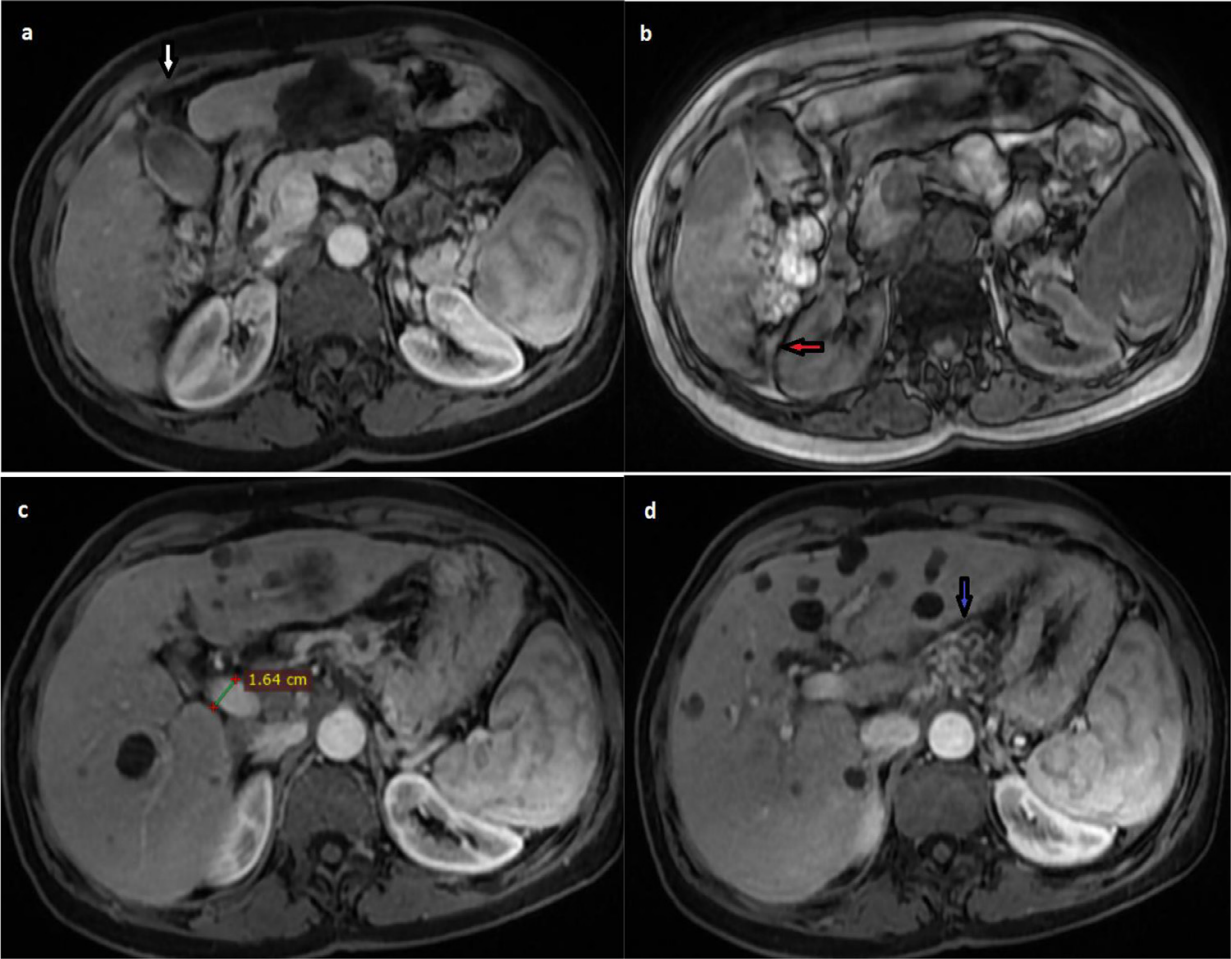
hepatic MRI on T1 post contrast WS (A, C, D) and OP WS (B) showing a cirrhotic liver with hypertrophy of the caudal lobe (note the right liver notch sign [red arrow), and atrophy of segment IV (white arrow), with portal hypertension (dilatation of the portal trunk and portosystemic collaterals [blue arrow]). (Color version of figure is available online)
